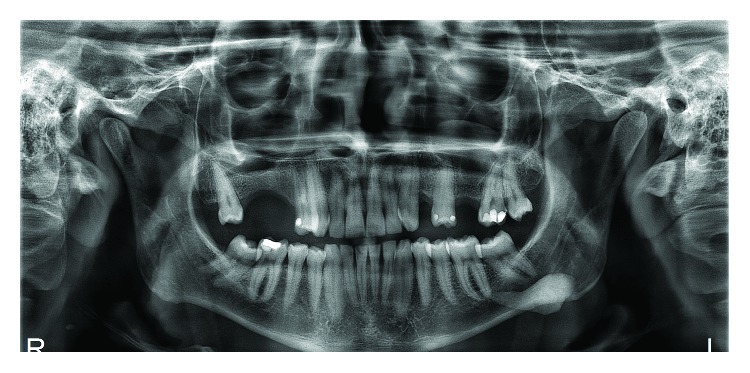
Panoramic radiography revealing radiopaque mass in the region of the left mandibular body.
